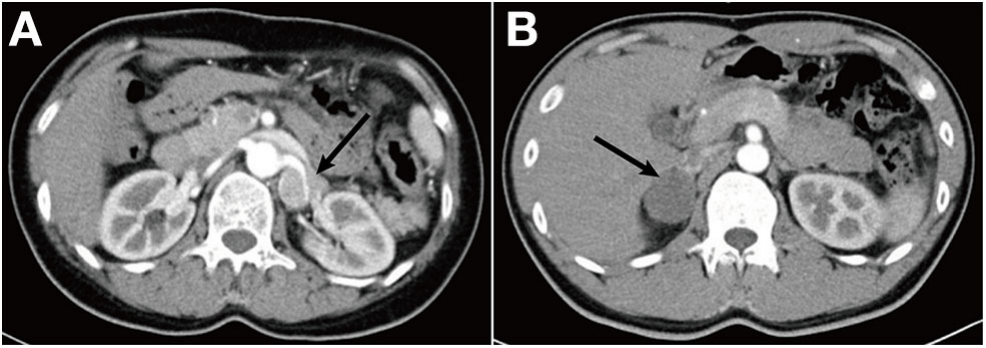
(A) Abdominal contrast-enhanced CT scan demonstrates an irregular left adrenal mass (4.1 × 2.7 × 3.3 cm) with a density of 42 Hounsfield unit (HU), wrapping the left renal artery. (B) CT scan showed a right adrenal mass (3.7 × 3.2 cm) with a density of 28 HU.
